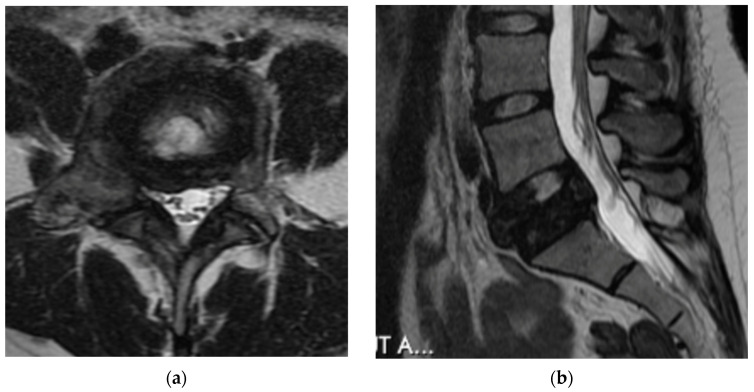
(a) L5 Giant Cell Tumor after 6 months of IV denosumab treatment. T2 weighted axial MRI cuts of L5 vertebral body show normalized bone marrow signal intensity when compared to pre-denosumab imaging. (b) Sagittal T2 weighted cuts of L5 Giant Cell Tumor after 6 months of IV denosumab therapy show reduction of retropulsion into the canal likely secondary to consolidation of tumor.
